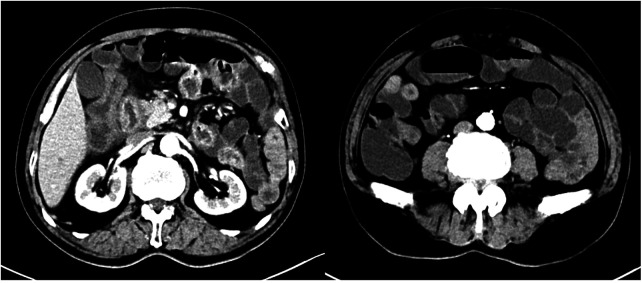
Abdominal CT scan showing paralytic ileus and duodenal edema.
